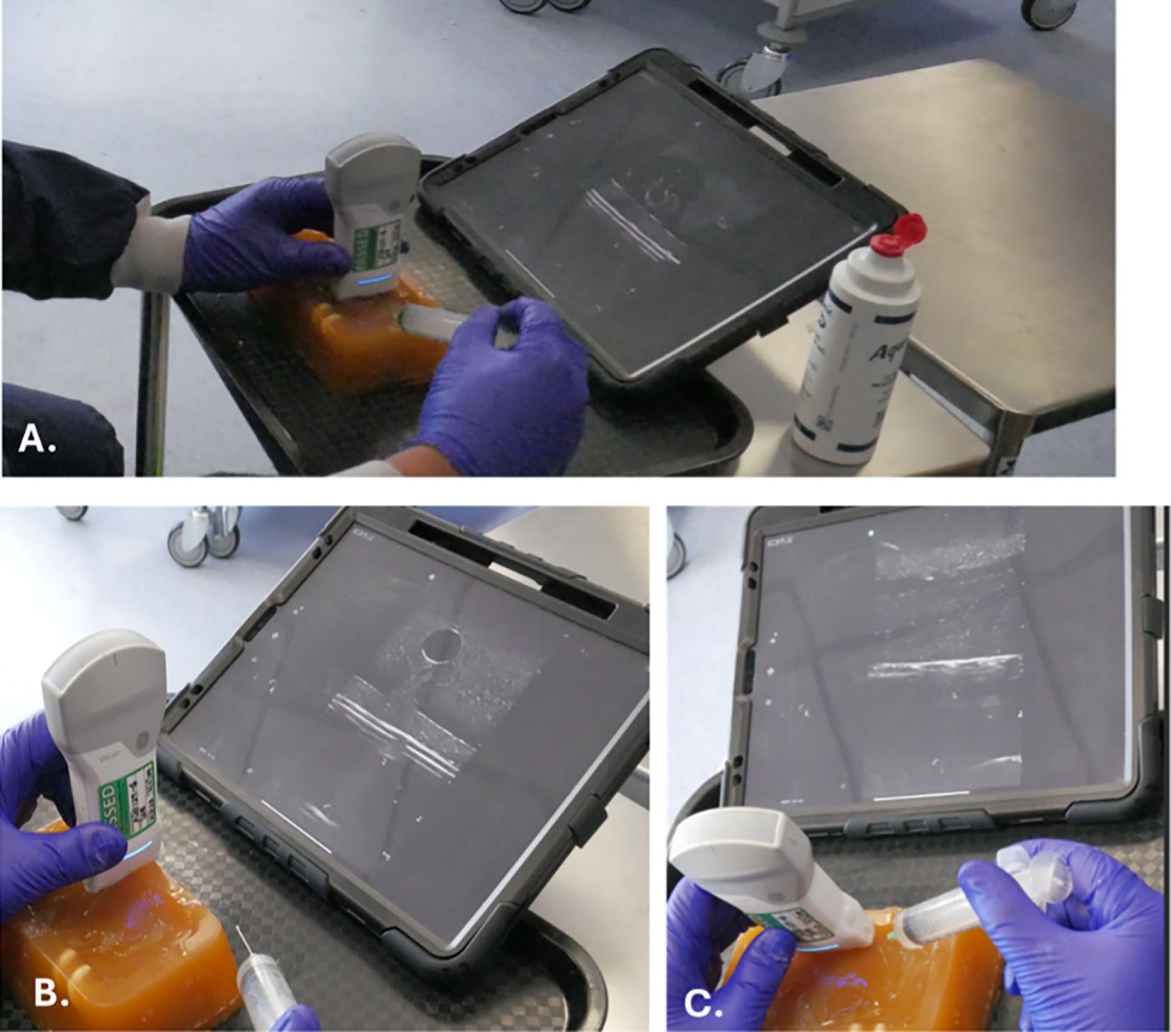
Set-up of the ultrasound gel training model for ultrasound-guided venepuncture, showing. A. General set-up, B. Out-of-plane venepuncture, and C. In-plane venepuncture.
